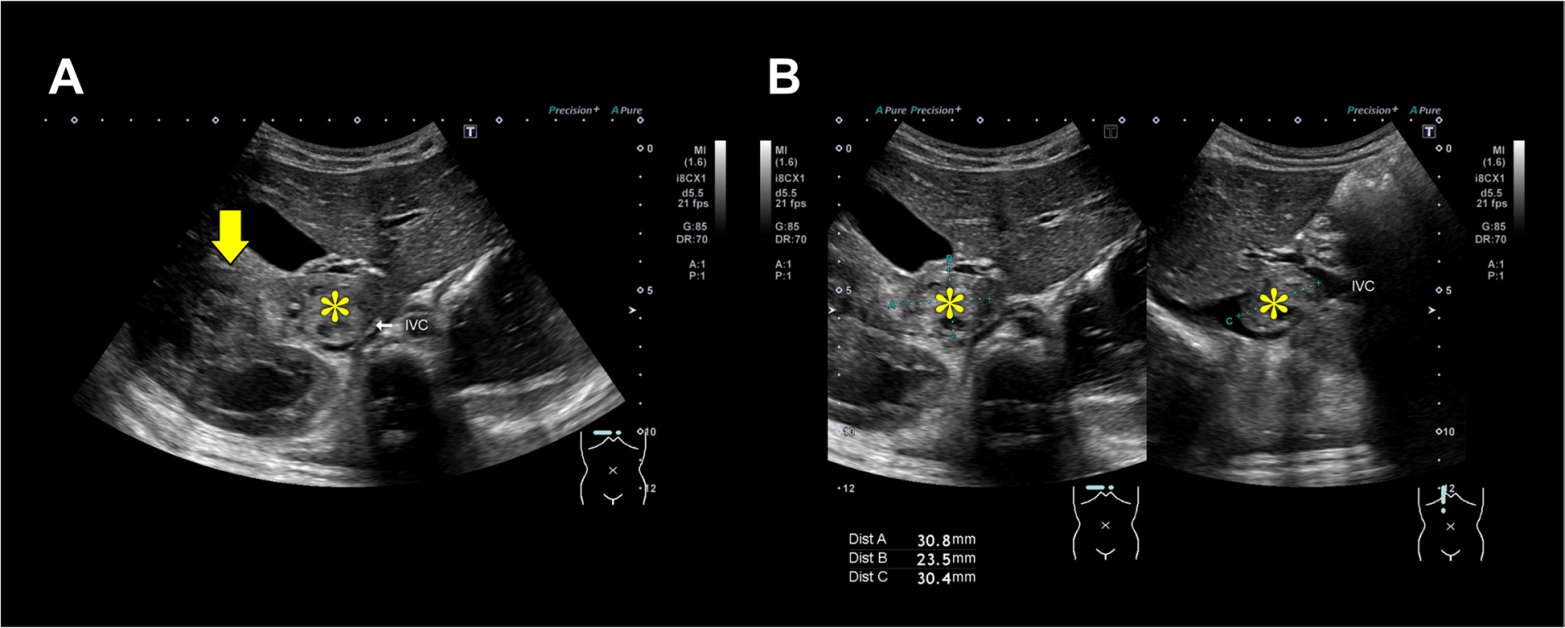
Abdominal ultrasonography. A Image demonstrating the right renal tumor (yellow arrow) and its intravascular extension into the inferior vena cava (IVC), marked with an asterisk. B Transverse (left) and longitudinal (right) ultrasonographic views of the tumor extending into the IVC, with the intravascular portion marked by an asterisk
